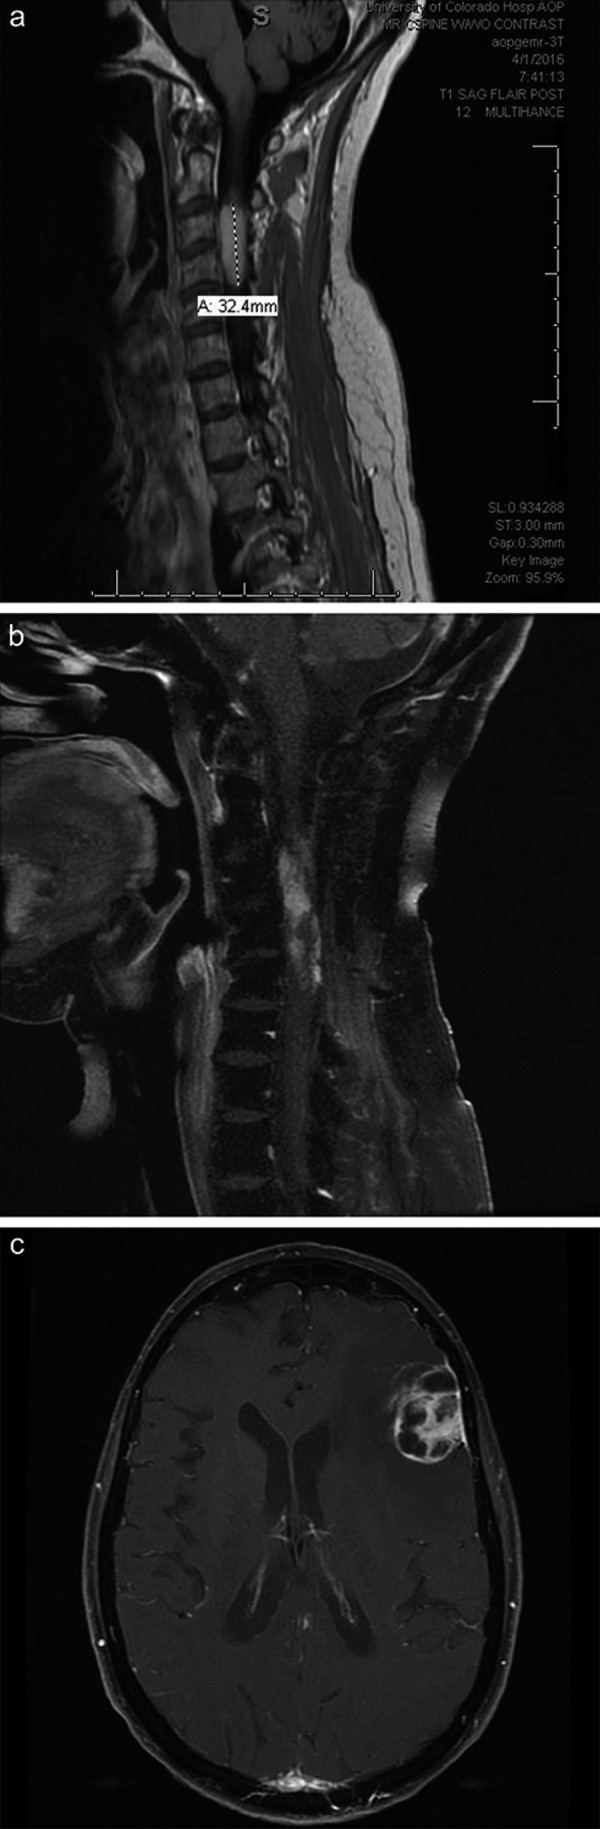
Aggressive nature of diffuse midline gliomas with *H3 K27M* mutation and pylotic astrocytome (PA) morphology, with later metastatic recurrence. a: Contrast-enhanced sagittal T1-weighted image demonstrating a cervical cord PA (patient 4) at presentation. b: Contrast-enhanced sagittal T1-weighted image demonstrating local progression within 1 year of diagnosis of the PA shown in (a). c: Contrast-enhanced axial T1-weighted image demonstrating additional large supratentorial leptomeningeal/dural metastasis with transformation of the PA to glioblastoma 1 year after diagnosis.
